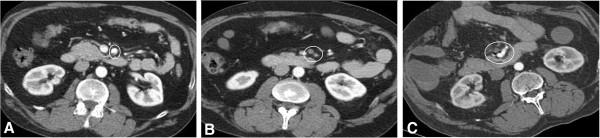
Representative CT scan findings. A: Dissection entry in the SMA at the typical location after passing behind the neck of the pancreas and the splenic vein. B: total occlusion of a branch of the SMA distal to the dissection entry. C: findings of the CT control 1 day after operation are shown. No residual membrane could be observed, normal perfusion of the SMA and the obstructed branch.
